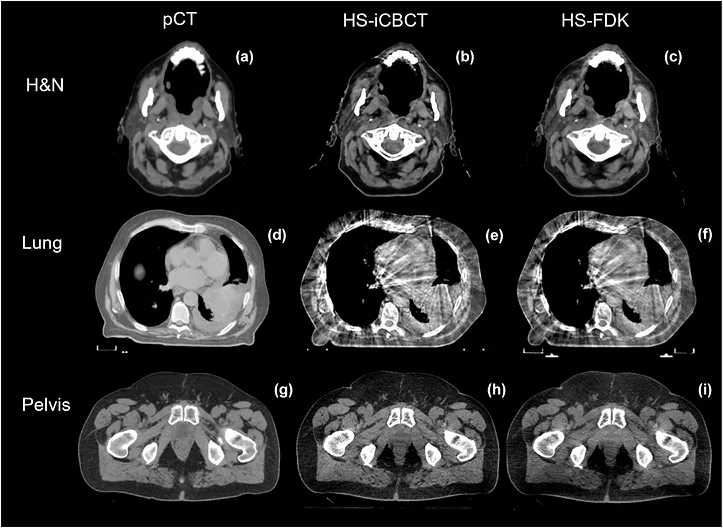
Patient image quality comparison between HS‐iCBCT and HS‐FDK reconstruction relative to the pCT for patient datasets acquired at a CBCT acquisition energy of 140 kV. (a–c) H&N patient, (d–f) Lung in free‐breathing and (g–i) Prostate patient.
